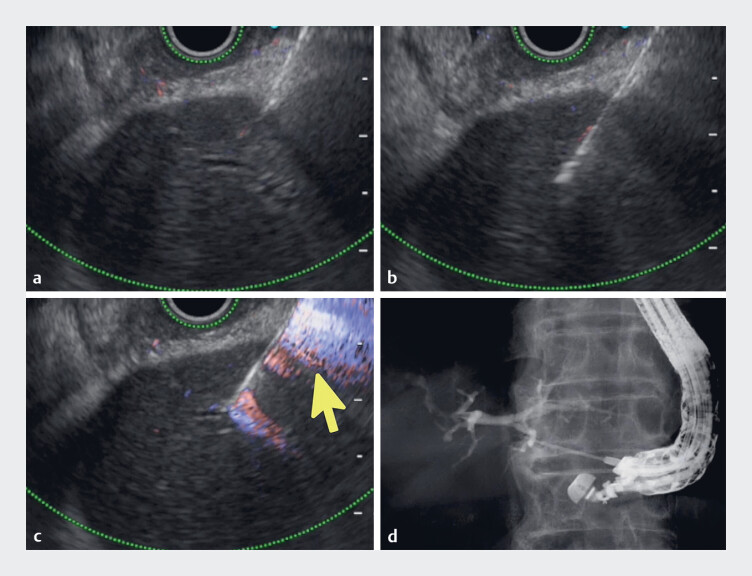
Images of endoscopic ultrasound-guided hepaticogastrostomy being performed in a patient with a nondilated bile duct showing:
